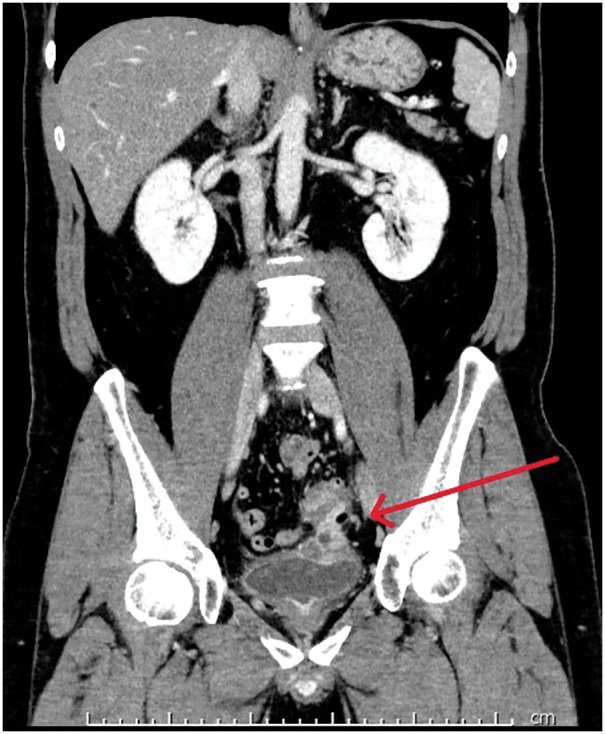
CT showed multiple diverticula in the sigmoid colon with increased density of surrounding fatty tissue. A colovesical fistula was formed. The arrow indicates the fistula that contacts the bladder dome, and the contrast enhancement extends continuously to the bladder mucosal surface. A small amount of air was noted within the bladder.
